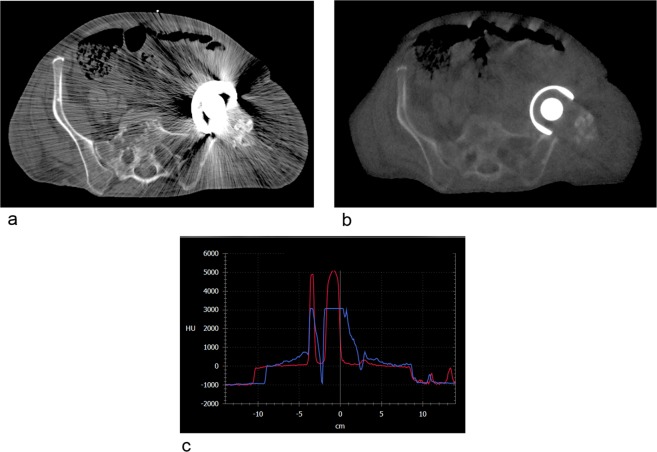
Differences in image quality for patients with metal implants using a kilovoltage CT (a) and the Radixact MVCT (b). Graphic (c) shows the Hounsfield unit (HU) comparison of image (a) in blue and image (b) in red. Obviously, densities with associated HUs above 3071 are not.
